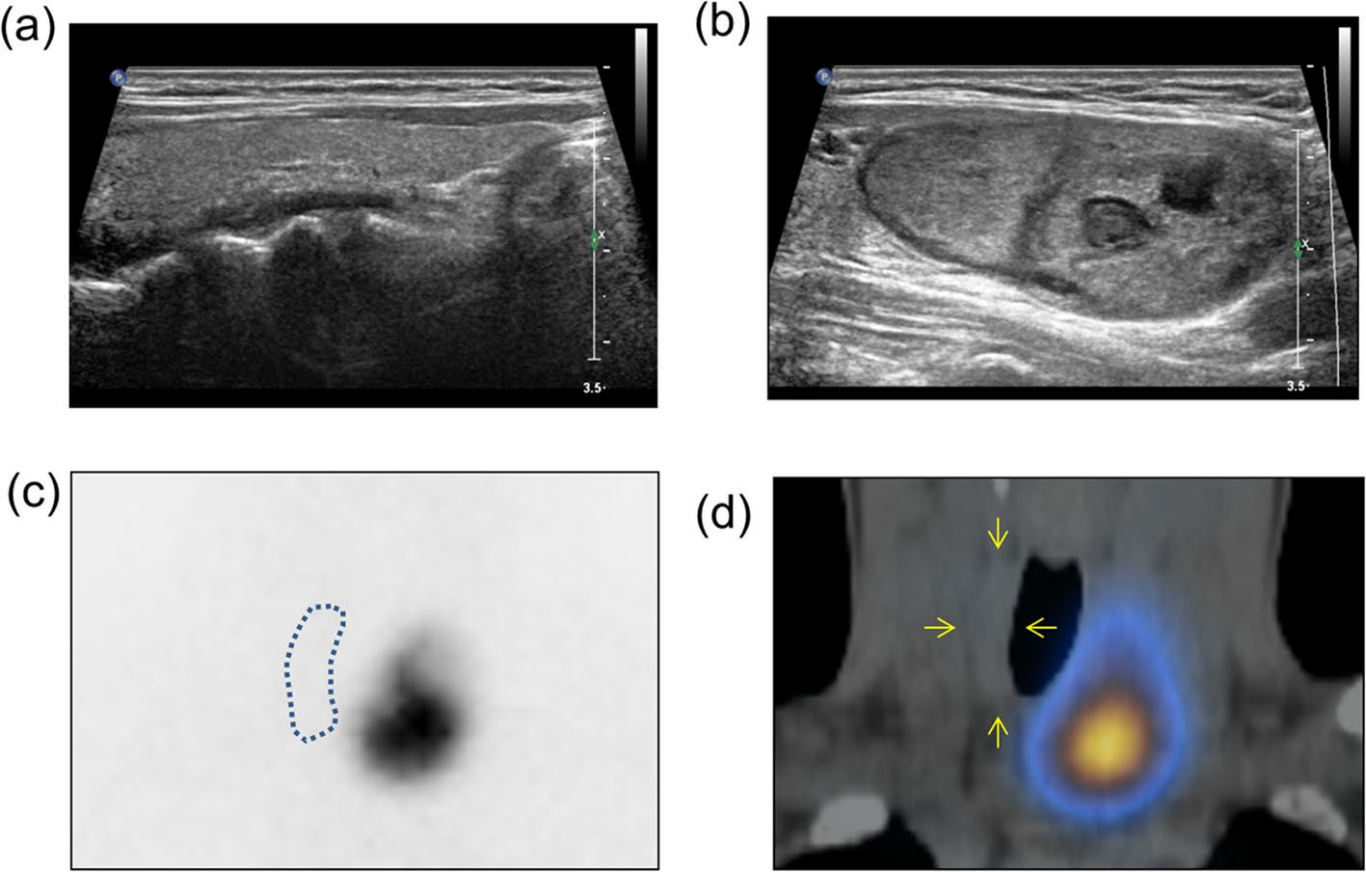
An autonomously functioning thyroid nodule. The neck ultrasound does not show abnormal findings in the right lobe (a), while a large thyroid nodule (3.44 × 2.14 × 4.84 cm) is observed in the left thyroid lobe (b). Tc-99m pertechnetate scan reveals a hot nodule in the left lobe, while the right lobe is suppressed, as demonstrated on the planar scan (dotted line) (c) and SPECT/CT (small arrows) (d). *SPECT* single-photon emission computed tomography
